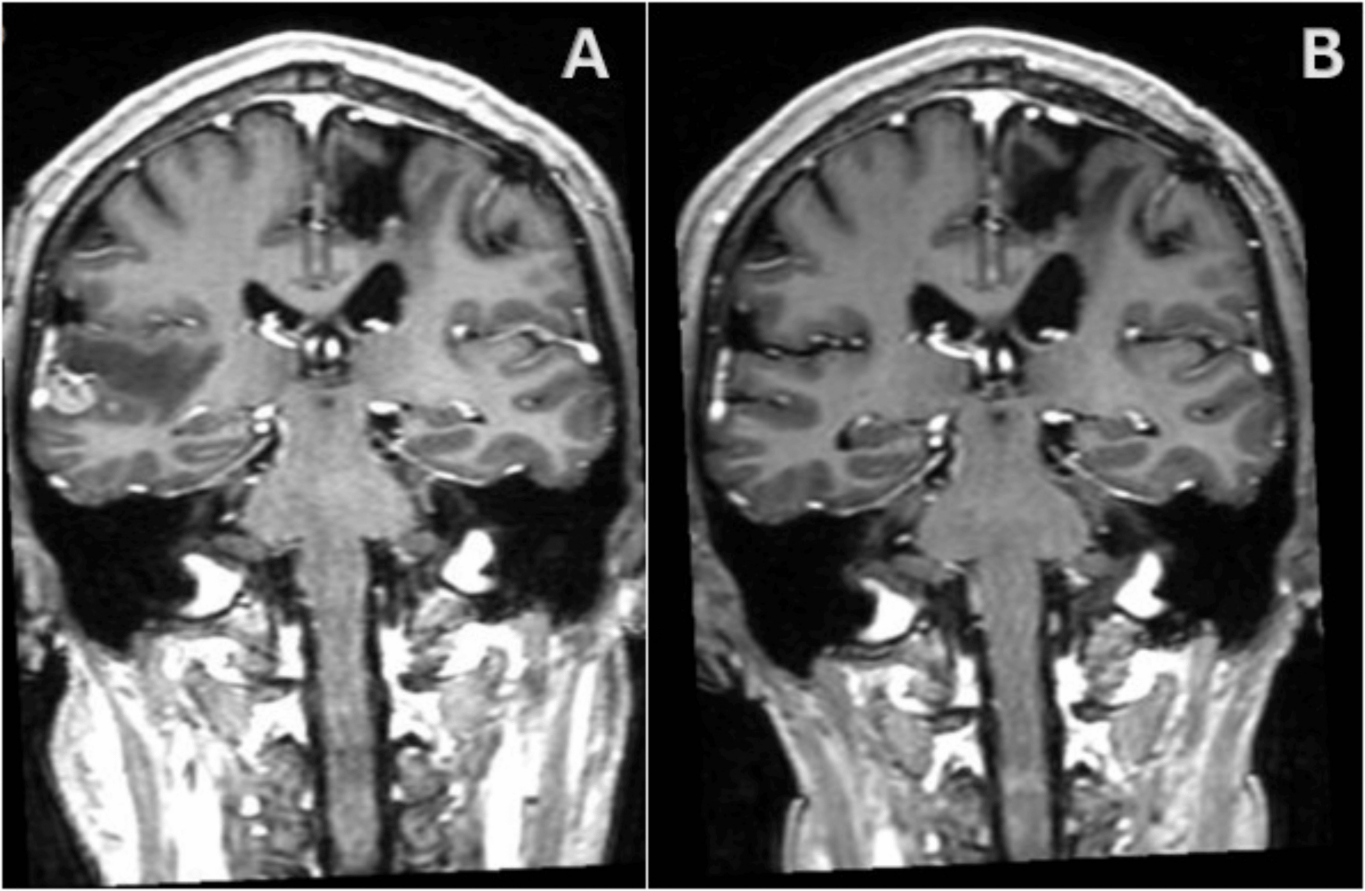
Patient B, pre- and post-GRS imaging (A) New 15 mm right temporal metastatic lesion on pre-Treatment axial T1 post-contrast MRI. (B) Complete response of the target lesion on post-treatment axial T1 post-contrast MRI. GRS: gyroscopic stereotactic radiosurgery
